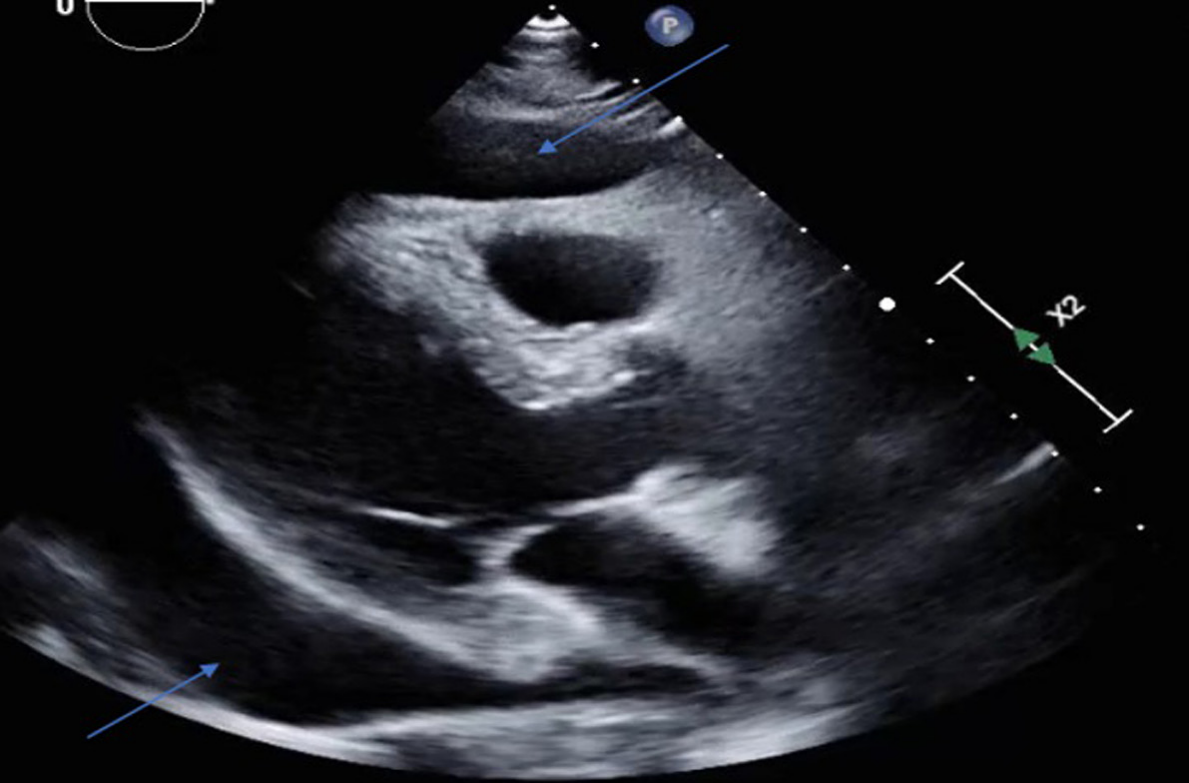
Transthoracic echocardiogram revealing large pericardial effusion.
